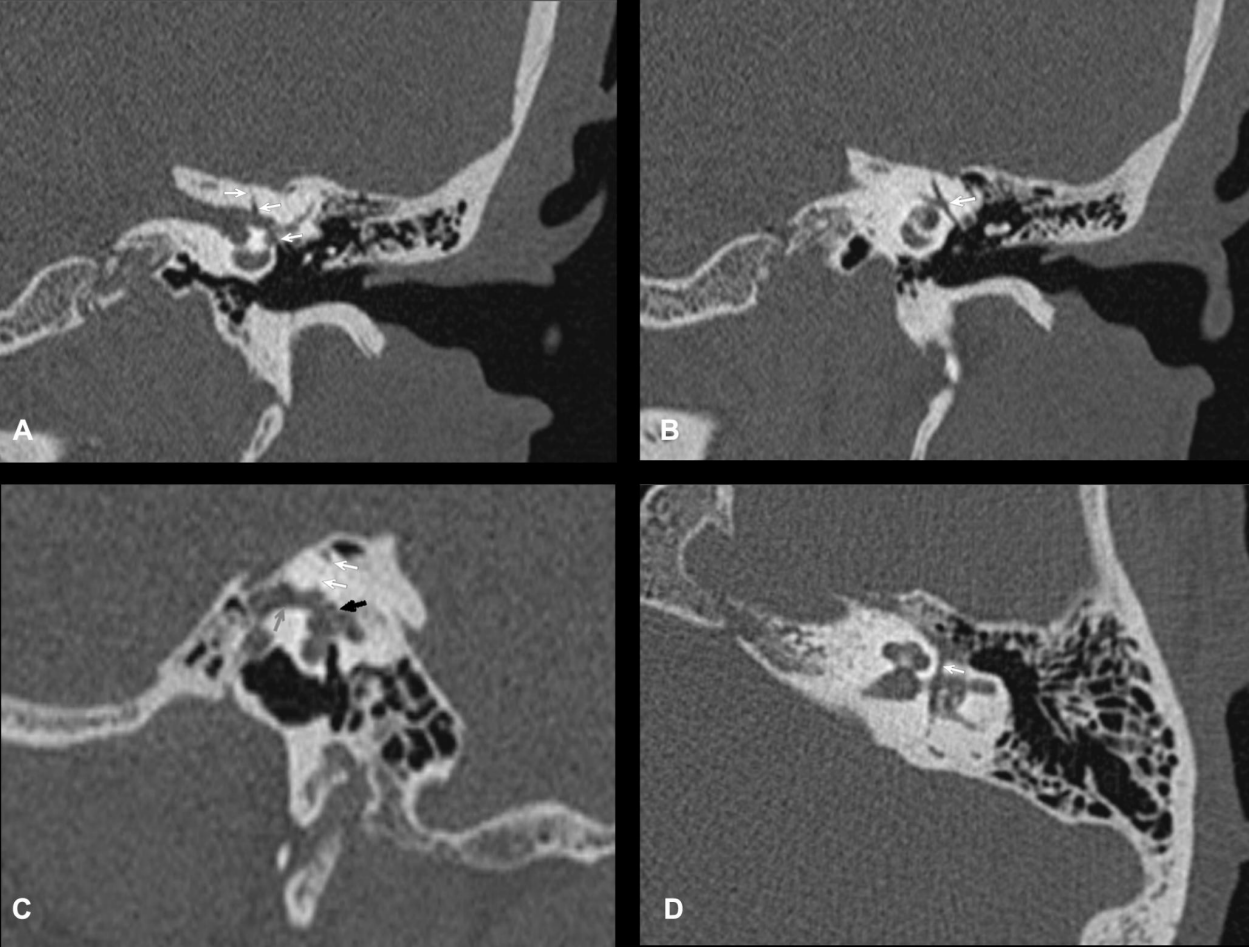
CT scans of the left inner ear. (A) coronal plane, white arrows present fracture affecting vestibule and internal acoustic meatus; (B) coronal plane, white arrows present the fracture, which is in close vicinity of the cochlea but is not damaging it; (C) sagittal plane, white arrows points at fracture, gray arrow points at internal acoustic meatus, black arrow: vestibule; (D) axial plane, fracture involves the vestibule
